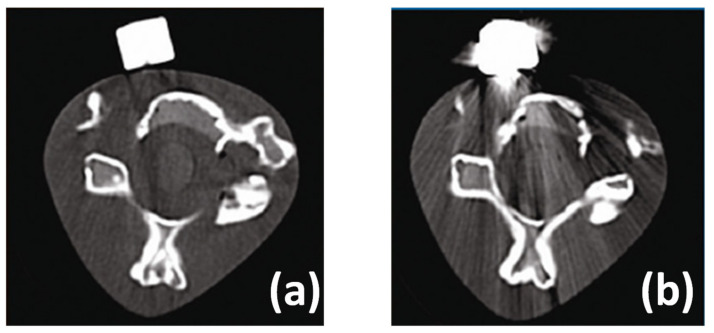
CT images (a) with a PEEK-OPTIMA image contrast grade and (b) with a metallic implant. Reproduced with permission from [46]. Copyrigth 2019, Elsevier.
